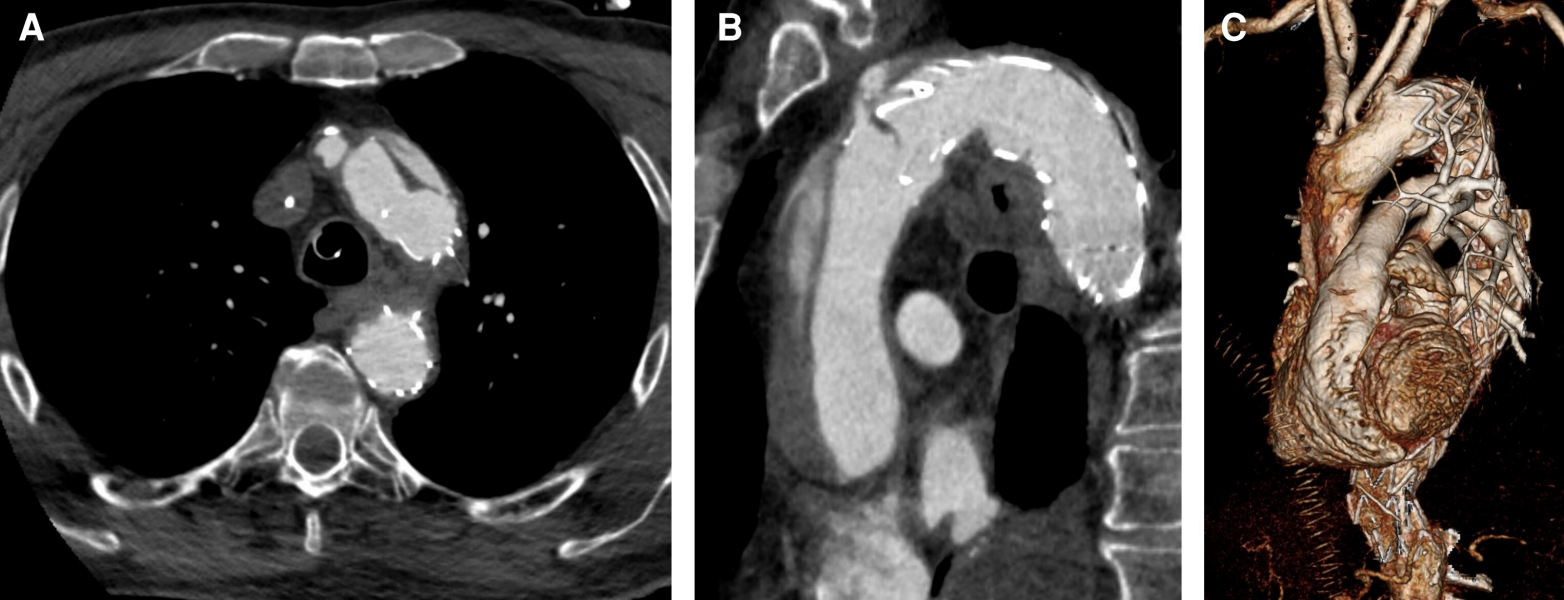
CTA after TEVAR and consecutive retrograde ATAAD. (A) Entry proximal of TEVAR stent graft. (B) Dissection involving the ascending aorta and the aortic arch. (C) 3D reconstruction.
